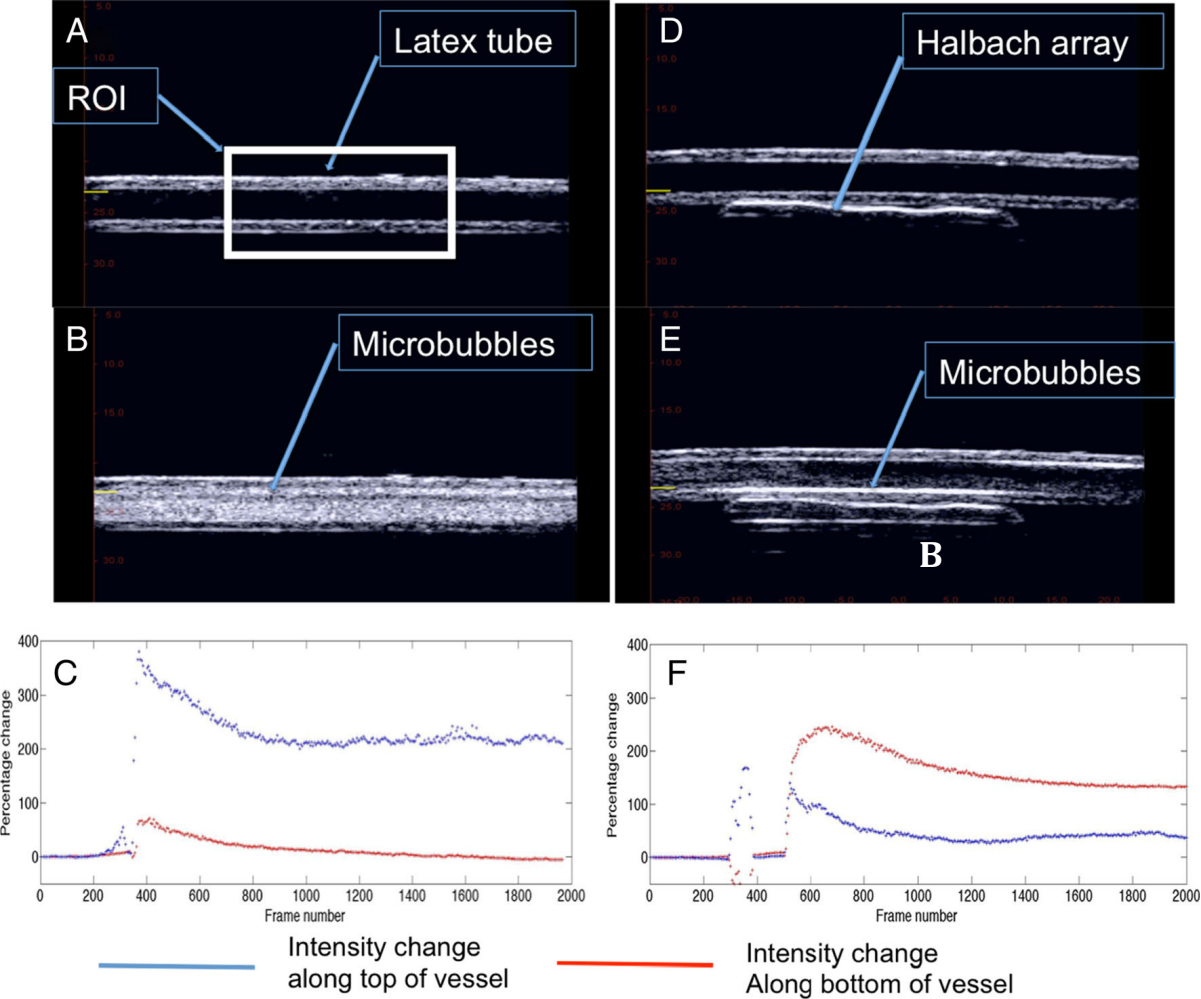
Acoustic images of targeting of magnetic microbubbles. a Latex tube with water flowing through. b After injection of magnetic microbubbles. c Intensity analysis in the region of interest (*ROI*) along the bottom wall (*red*) of the vessel and the top wall (*blue*) indicates the highest signal intensity was detected at the top of the tube over the course of the ultrasound. d The same latex tube with a magnetic Halbach array positioned underneath with water flowing through. e After injection of magnetic microbubbles, an increase in signal intensity is observed along the bottom of the tube. f Intensity analysis within the same ROI along the top (*blue*) and bottom (*red*) walls of the tube indicates that the highest intensity occurred at the bottom of the tube at the wall closest to the magnetic Halbach array
